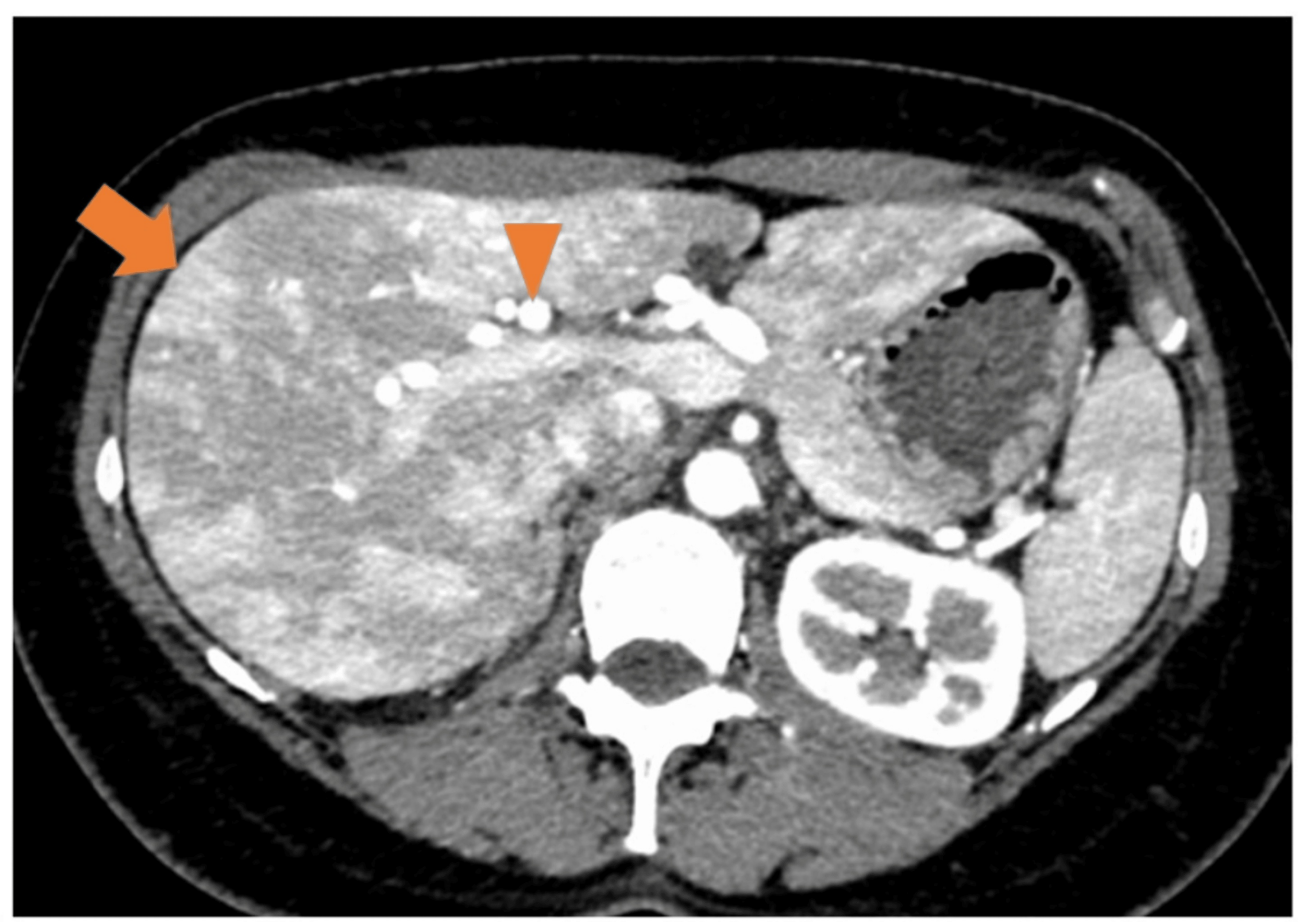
Early phase of dynamic liver computed tomography (CT) shows a wedge-shaped enhancement (arrow) and hepatic artery dilatation (triangle).
